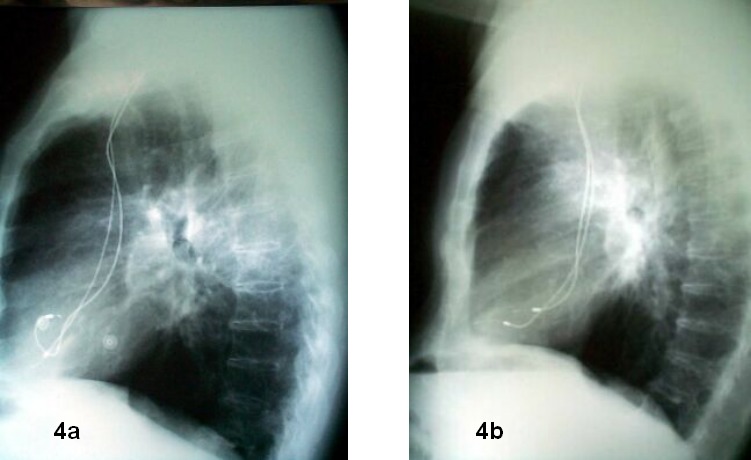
Lateral chest x-ray film (same patient as in figure 3): left (A): 24 hours post implantation. Right (B): 3 months later. Atrial lead displacement diagnosis is now straightforward. The example emphasizes the relevance of two chest views available to correctly evaluate lead displacement.
